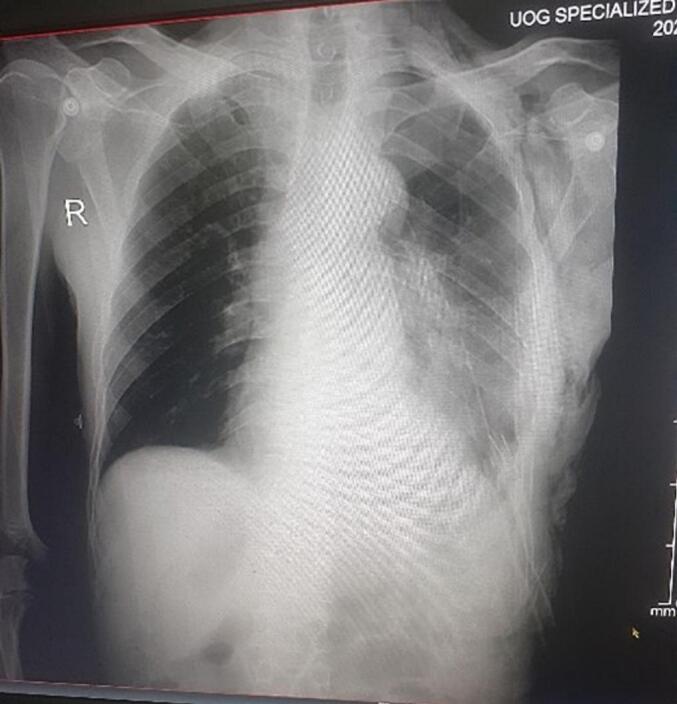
Postoperative chest X-ray on day 2 showing lung expansion.
